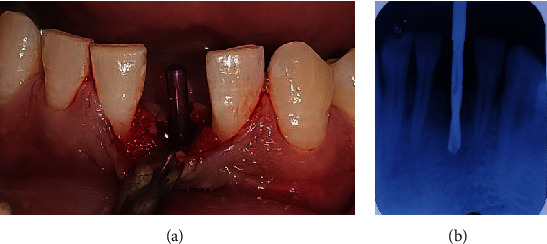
(a) Paralleling pin placement. (b) Intraoral periapical radiograph with guide pin.
